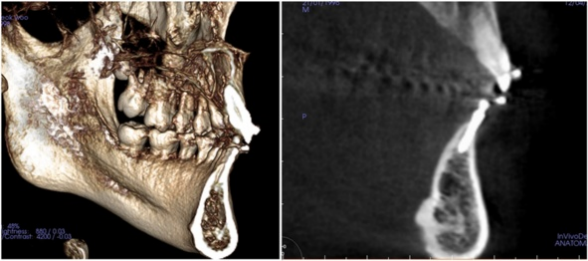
Post treatment CBCT view. Because buccal plate of alveolar bone was concave, buccal placement of C-implant was inevitable.
